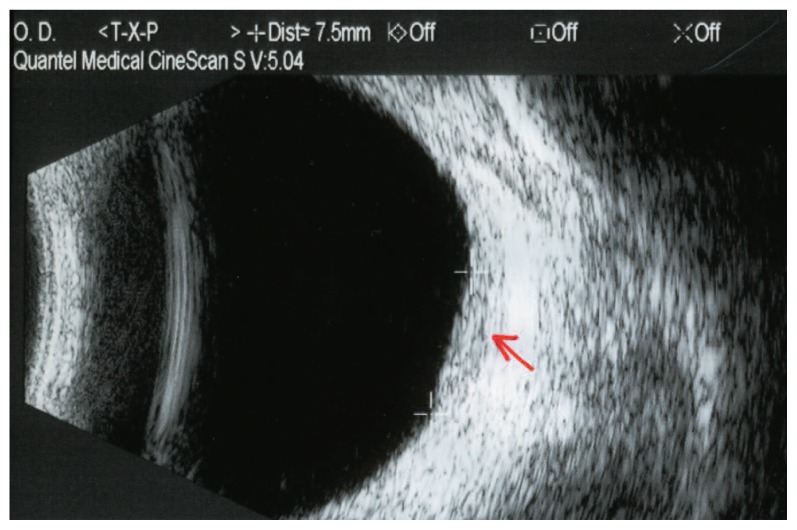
Ultrasound scan of the same eye as in figure 1 before treatment (March 9, 2007); a choroidal mass 8.8×7.5 mm in basal dimensions and with maximal elevation of 1.74 mm is apparent in the temporal aspect of the globe (arrow).
